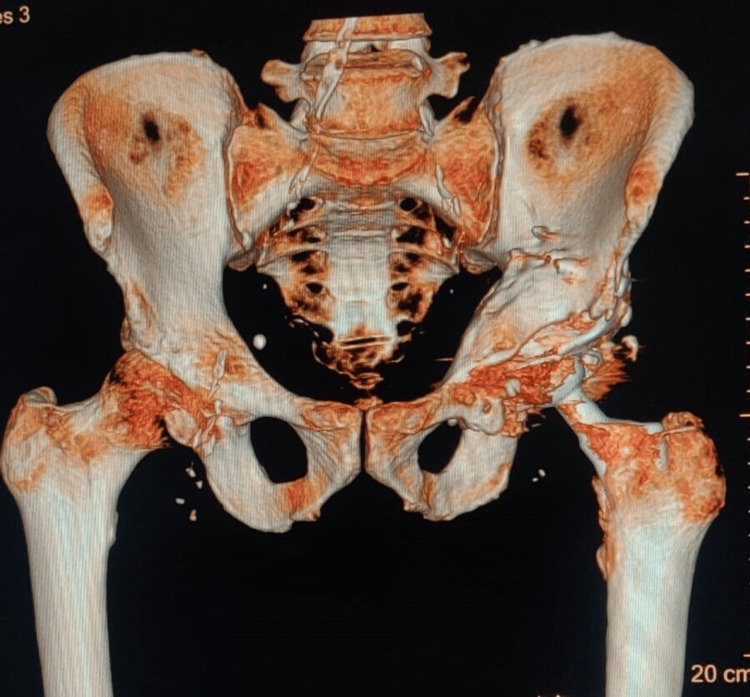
CT scan of the hip during the 12-month follow-up visit showing the union of both columns
